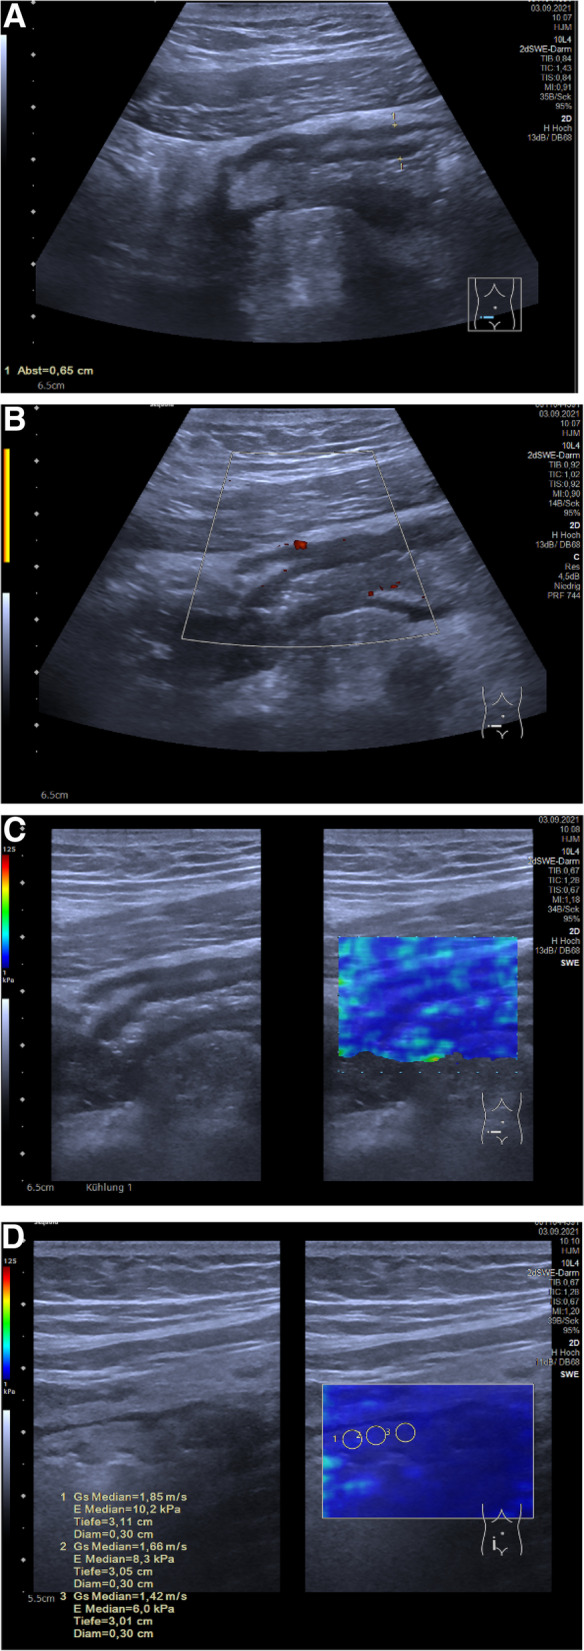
A 16-year-old male with stenosing ileitis in Crohn’s disease. Ultrasound in routine follow-up without acute problems or increasing clinical score. A B-mode image revealing increased bowel wall thickness (6.5 mm) (Sequoia, Siemens, L10-4 probe). B Power Doppler imaging without any pathological increase in vascularization (Sequoia, Siemens, L10-4 probe). C SWE with no pathological qualitative distribution in color scale (Sequoia, Siemens, L10-4 probe). D SWE using quantitative evaluation with three regions of interest within the submucosa of the small bowel (median 1.42, 1.66, 1.85 m/s) (Sequoia, Siemens, L10-4 probe) which is lower than published cutoff values for active inflammation [52]
